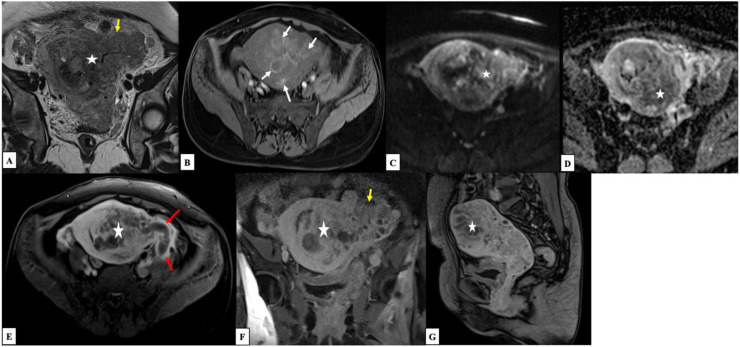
Axial MRI shows a substantial anterior myometrial tumor with irregular margins (star), exhibiting variable T2-weighted hyperintensity (A) with hemorrhagic areas showing high T1 signal intensity (white arrows), central necrotic areas, and significant restricted diffusion on diffusion-weighted imaging (C) with low ADC values (D). Post-contrast sequences demonstrated intense, heterogeneous enhancement (E, F, G). The tumor showed extensive local invasion into the endometrium, exocervix, the upper two-thirds of the vagina, and the parametria. Notably, an extraserosal extension was identified on the left (yellow arrow), invading the adnexa and resulting in tumor thrombosis of the left ovarian vein (red arrow).
